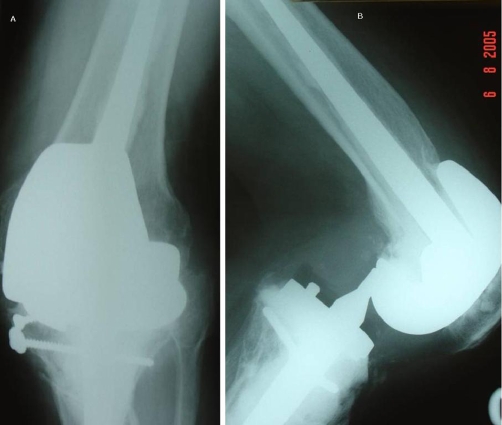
(A) AP x-ray of the revision knee arthroplasty at dislocation. (B) Lateral x-ray of the revision knee arthroplasty at dislocation (showing the “Cam jump”).
